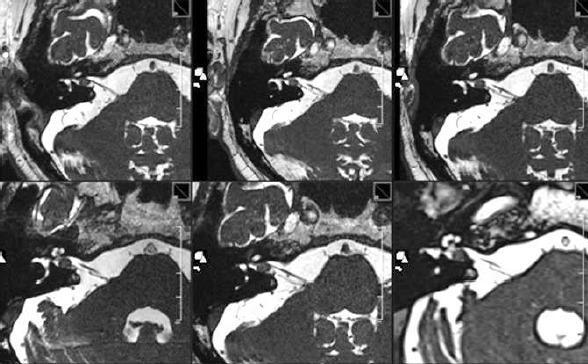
MRI 1.5 T; CISS axial; 0.8 mm slice thickness. Upper row: 5 years after diagnosis; 4 years after diagnosis; 3 years after diagnosis. Lower row: 2 years after diagnosis; 1 years after diagnosis; initial diagnosis.
